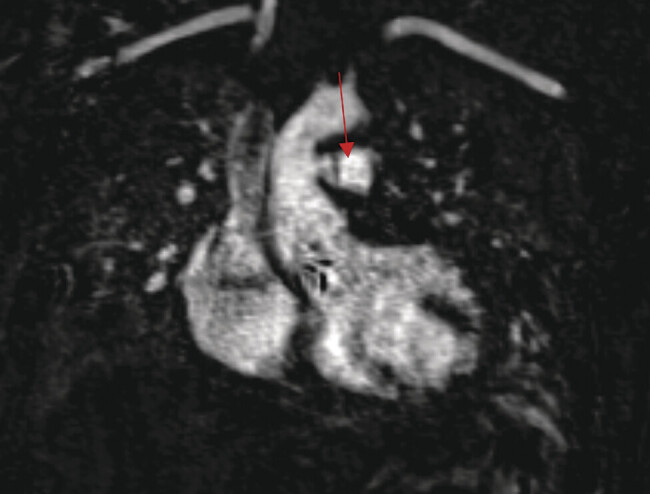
Cardiac MRI revealing the aortopulmonary connection. MRI, magnetic resonance imaging.
